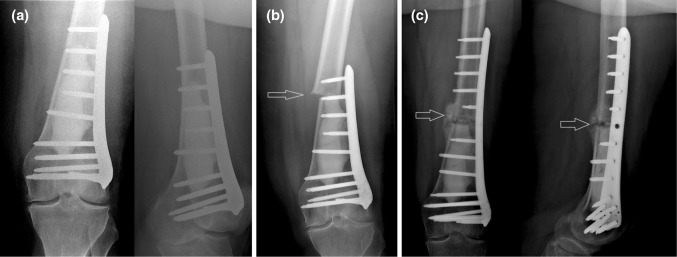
X-ray immediately after intralesional excision and osteosynthesis (a) followed by early fracture at the proximal part (red arrows) of the bone cement (b). Revision surgery with re-osteosynthesis with a longer locking compression plate resulted in callus bone healing (red arrows) 3 months after fracture (c) (color figure online)
